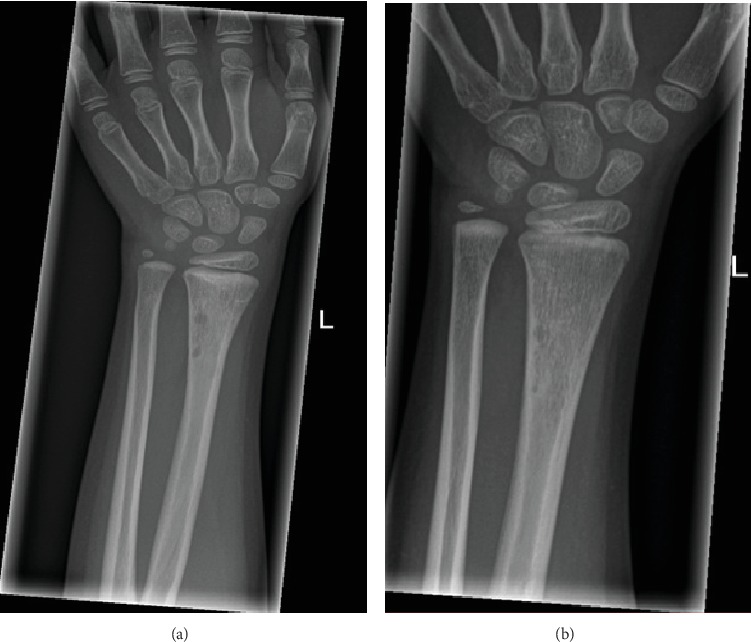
(a) Radiograph showing complete bone healing of the fracture. Two well-circumscribed lucent lesions in the cortex proximal to the previously fractured region. (b) Radiograph showing the lucent lesions fading away.
